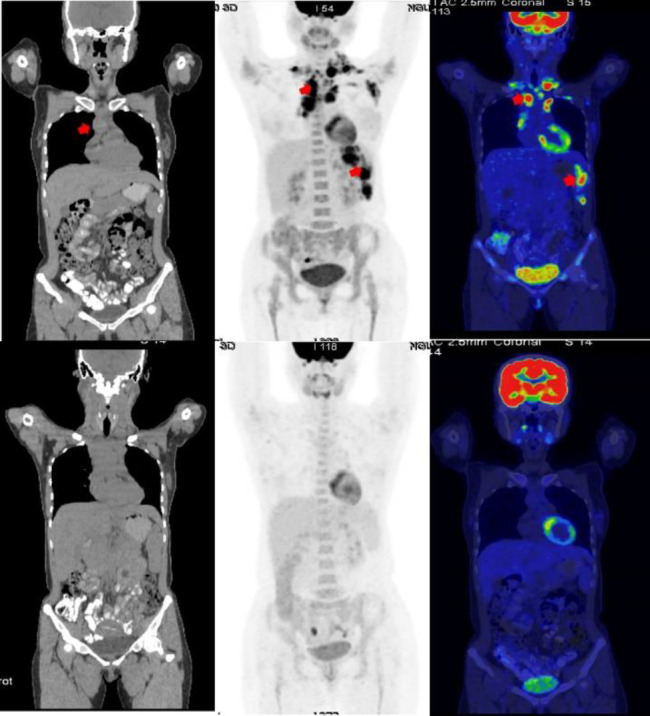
A 34-year-old female with stage IIIS mixed-cellularity classical Hodgkin lymphoma (cHL) presented with FDG-avid cervical/mediastinal lymph nodes (SUV_max_: 12.1) and splenic involvement (SUV_max_: 17.2) on baseline PET/CT (A-C, red arrow). After 2 cycles of ABVD, interim PET/CT revealed complete metabolic resolution (Deauville score 1), confirming complete metabolic response (CMR) (D-F). Treatment continued with 4 cycles of ABVD, achieving CMR with no recurrence on follow-up
